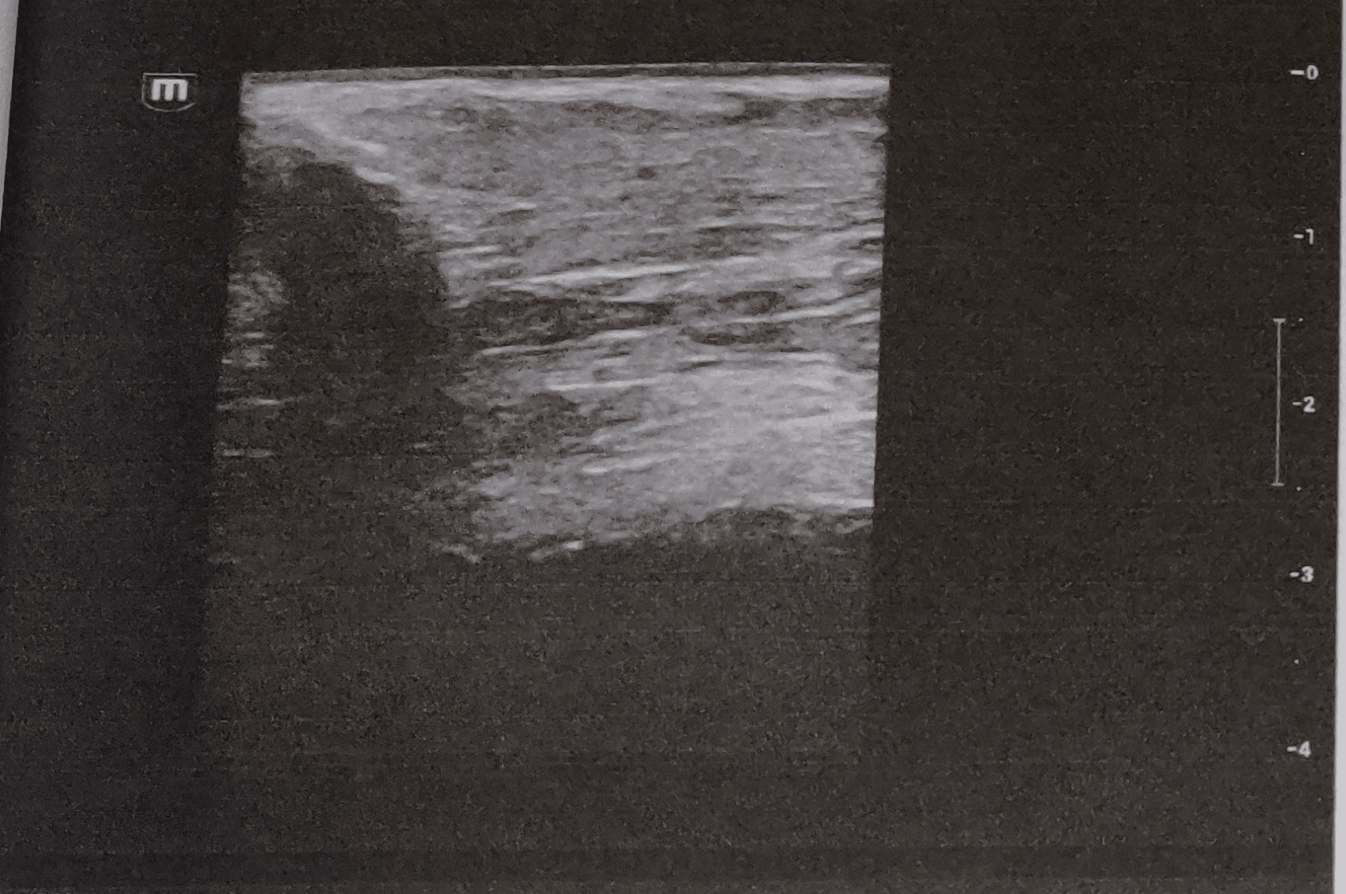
Intimal thickening on carotid Doppler ultrasound.
